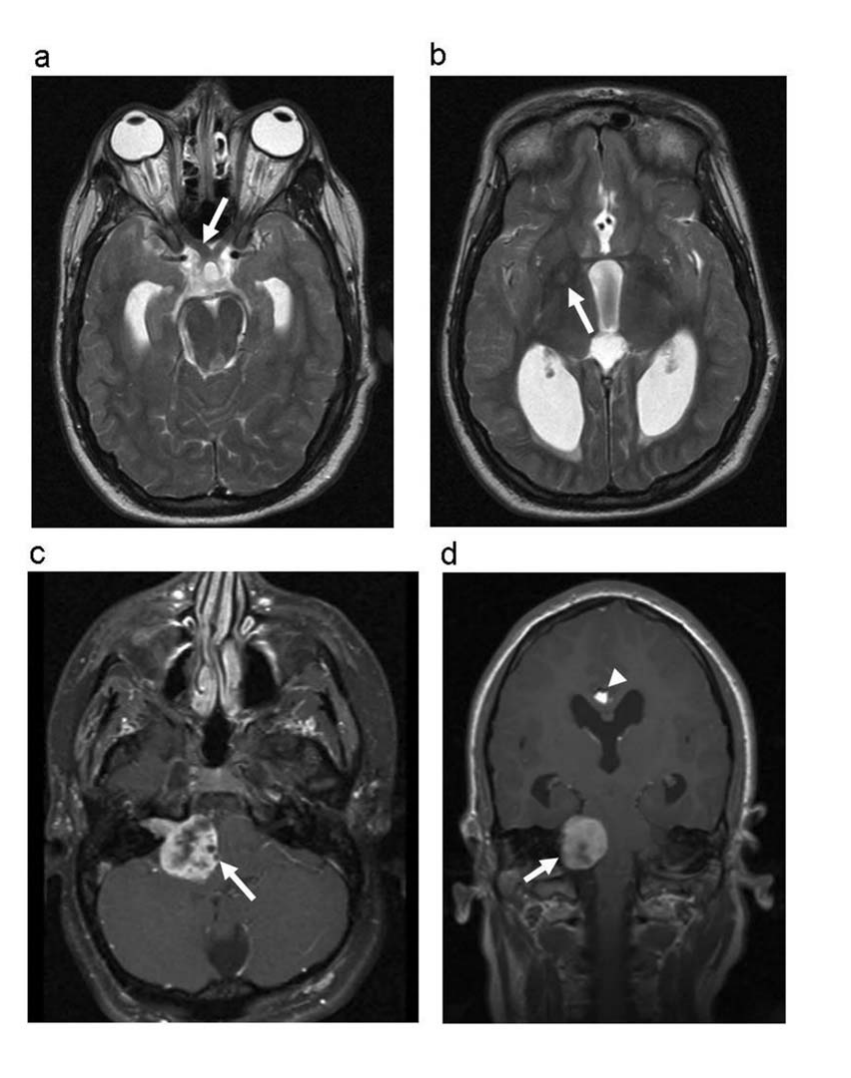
MRI images of the brain at the age of 20 years. (a) Horizontal T2 weighted section showing mild enlargement of the right optic nerve (white arrow) (b) Horizontal T2-weighted image showing a small hyper-intense spot in the right globus pallidus (arrow). Note also hydrocephalus. (c-d) Horizontal and coronal T1 weighted images showing the large partly cystic contrast enhancing schwannoma in the right cerebello-pontine angle. The tumoural mass causes deviation of the brain stem and the cerebellum to the left. (arrow) Note also supratentorial hydrocephalus and an interhemispheric lipoma (arrowhead).
